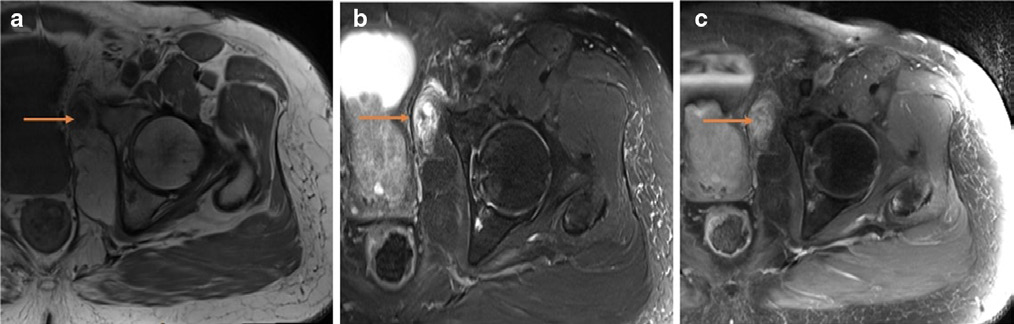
(a – c) MRI: (a) Axial *T_1_*-weighted, (b) fat suppressed *T_2_*-weighted and (c) fat suppressed *T_1_*-weighted with contrast demonstrating solid nodule that is low T1, high T2 with solid heterogeneous enhancement (orange arrow); imaging features supportive of an aggressive lesion. Orange arrows demonstrating abnormal aggressive features.
